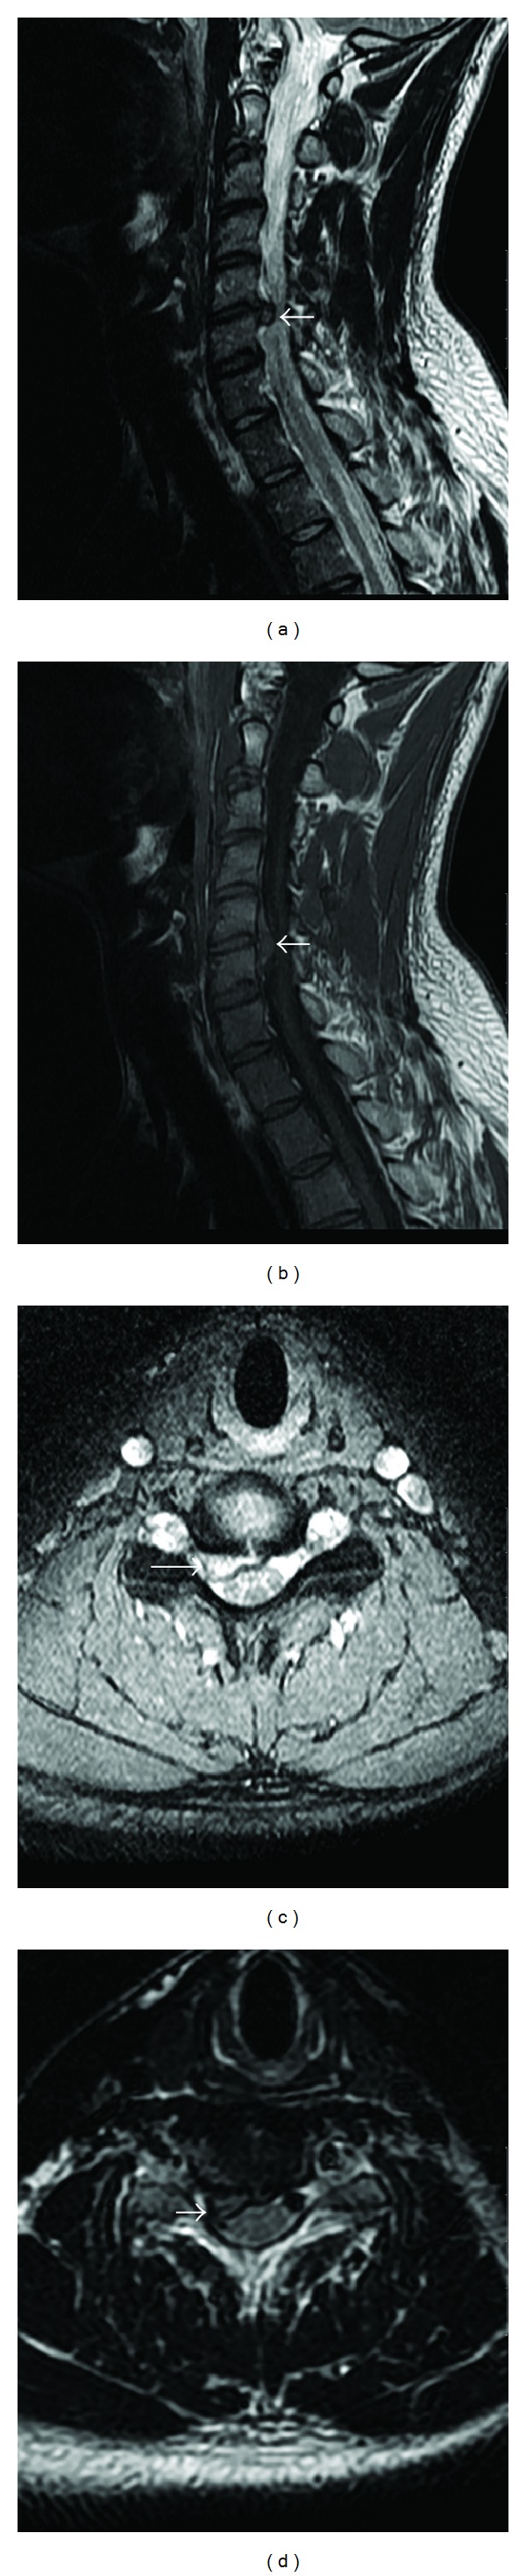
Sagittal T2-weighted (a), sagittal T1-weighted (b), axial T2* (c), and axial T2-weighted (d) MRI images show a large cranially and caudally migrating posterior disc extrusion (herniation) at C5-C6 level (arrows), eccentric to the right side and impinging upon the subarachnoid space, the cervical cord, and the right C6 nerve root.
